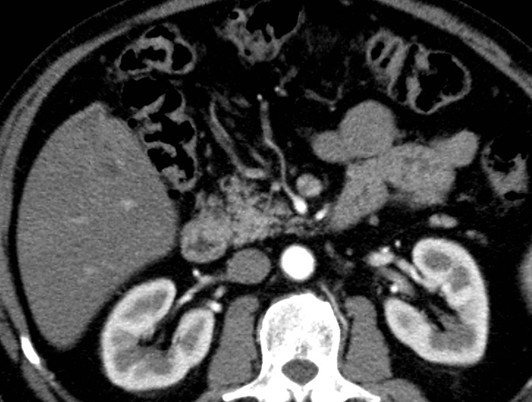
Transverse CT scan. It reveals a solid focal mass, about 3 cm in diameter, hypodense respect to the surrounding tissue in the basal phase (1) with a light peripherical enhanced rim in the arterial phase (2) that increases in the portal venous phase (3). In late phase (4), the lesion shows a puntacte aspect because of the presence of intralesional hypervascular spots (arrow). The bile ducts are not dilatated.
